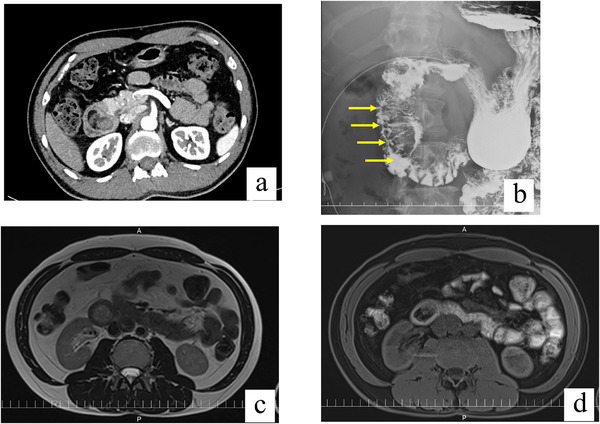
(a) Contrast‐enhanced computed tomography (CE‐CT) showing a mass lesion with mixed fat and soft‐tissue density extending from the duodenal bulb to the descending portion of the duodenum. (b) Upper gastrointestinal series showing an elevated lesion (arrow) measuring approximately 50 mm on compression views. (c) T1‐weighted magnetic resonance imaging (MRI) revealed a well‐defined mass with iso‐ to slightly high‐signal intensity. (d) T2‐weighted MRI revealed a well‐defined mass with moderately high‐signal intensity and areas of signal void.
